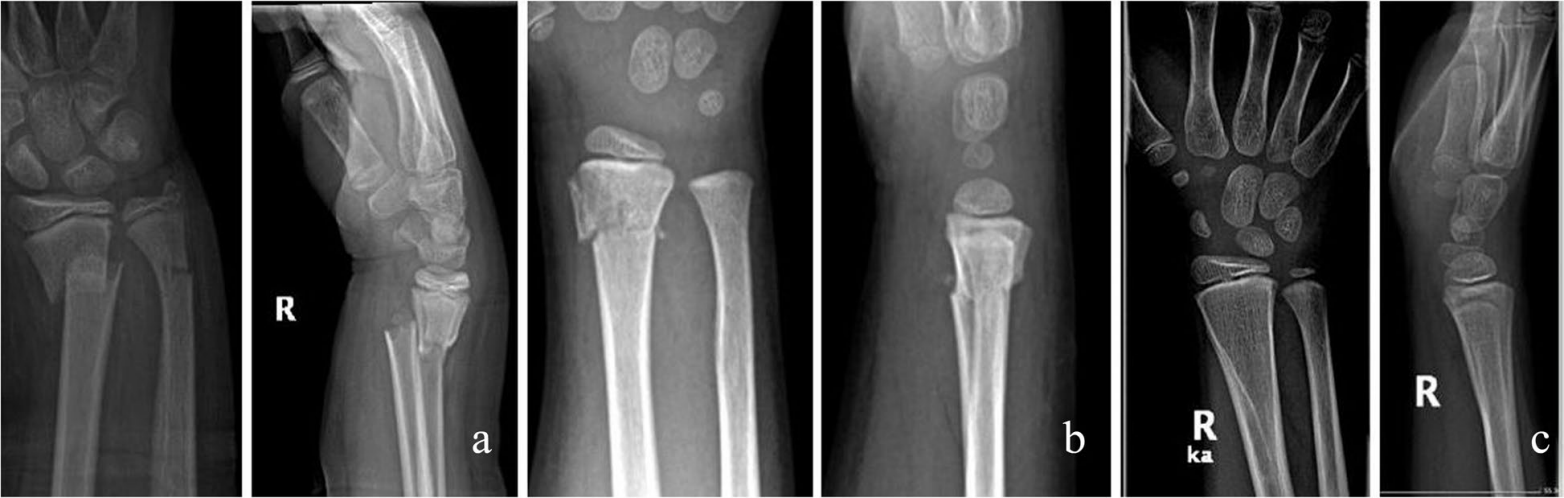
Distal forearm fracture of a 7-years old boy treated with Activa Pin™ a: shortened and displaced unstable distal metaphyseal fracture b: X-rays made in the postoperative first day c: X-rays made 24 months after surgery
